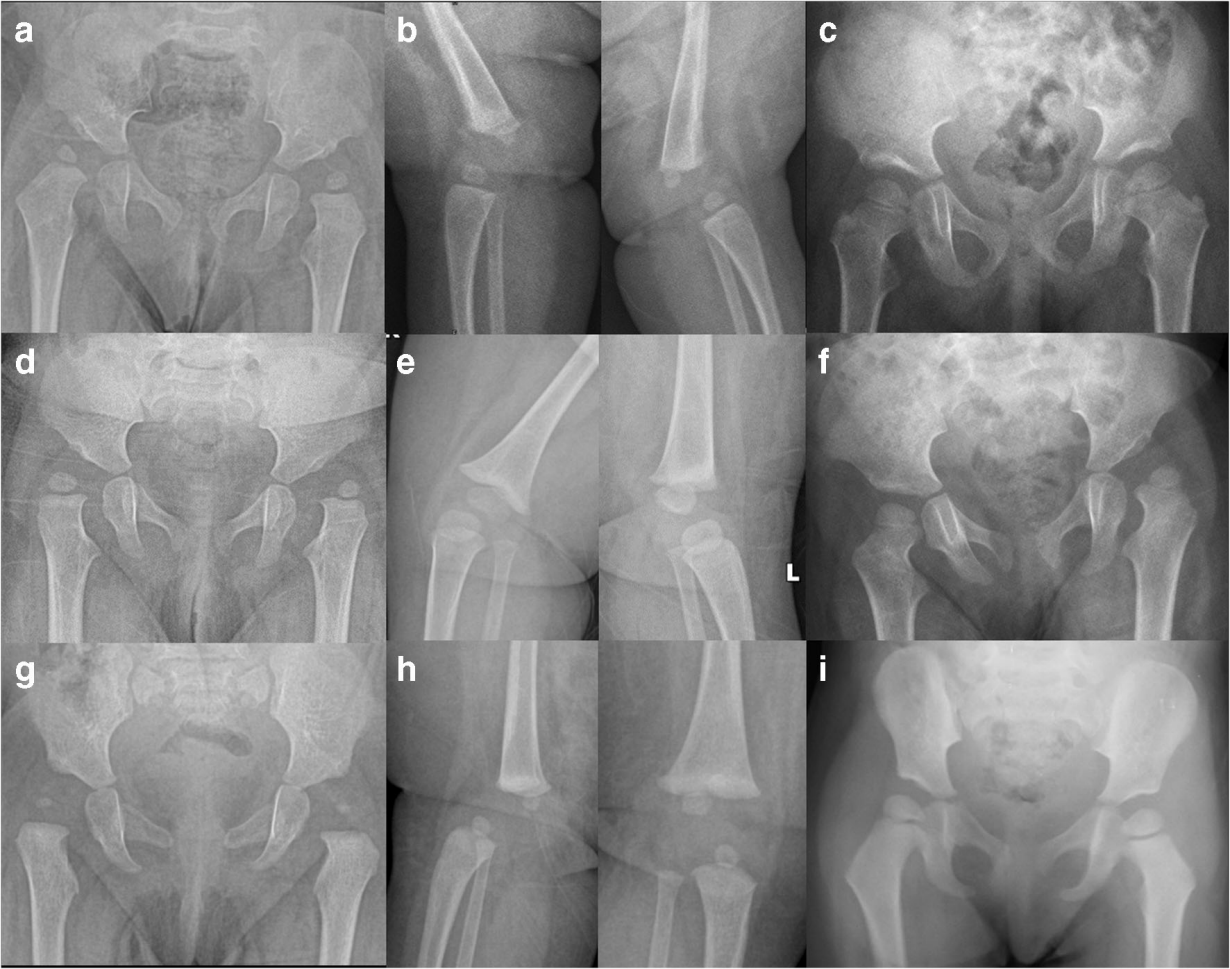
Nine-month girl (case 1) brought with bilateral congenital dislocation of the knee and bilateral CDH (a, b). She underwent bilateral quadricepsplasty. Radiograph taken at 3.5 years of age showed a spontaneous reduction of both the hip joints (c). Another 9-month girl (case 2) brought with bilateral congenital dislocation of the knee and CDH (d, e). She underwent bilateral quadricepsplasty. Radiograph at 15 months follow-up showed a spontaneous reduction of the right hip joint (f). The 6-month girl (case 3) presented with bilateral CDH and CDK (g, h). She underwent bilateral quadricepsplasty. Radiograph at 2-year follow-up showed a spontaneous reduction of both the hip joints (i)
